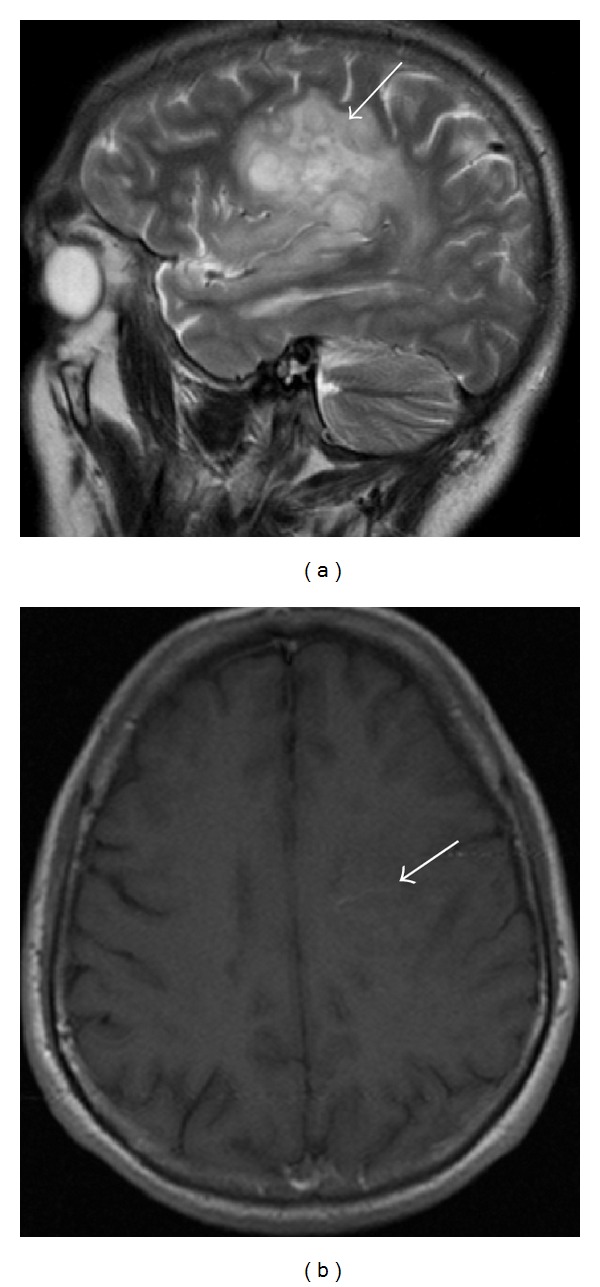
Cerebral MRI (2008): (a) large lesion in the left frontal lobe (large arrow). (b) Subtle contrast-enhancement (small arrow) and mass effect, suggesting an infiltrative lesion from glial series.
